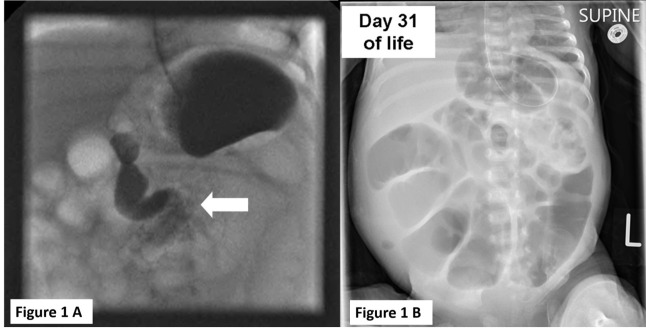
Figure 1: (A) Upper gastrointestinal contrast study performed on Day 4 of life showing D-J flexure position low and at the midline (arrow). (B) X-ray of the Abdomen showing persistent dilated loops 3 weeks post-Ladd procedure.
